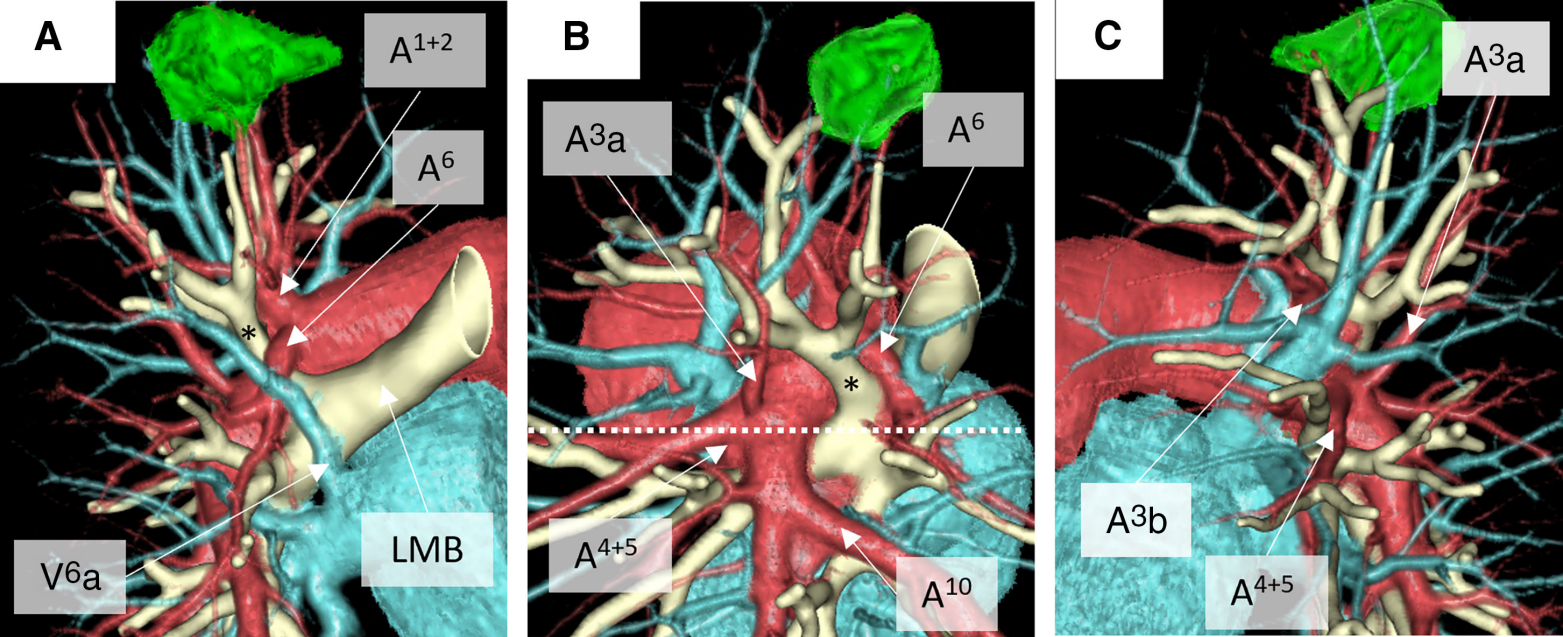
Preoperative 3-dimensional computed tomography reconstruction. Clockwise from the posterior (A) to the anterior (C) view. A left eparterial bronchus (*), or displaced left upper division bronchus (B^1+2+3^), with the pulmonary artery running anteriorly to the LMB. (B) A white dotted line shows the level where the left pulmonary artery crosses the LMB. A left eparterial bronchus originates from the LMB above the white dotted line. LMB, left main bronchus
